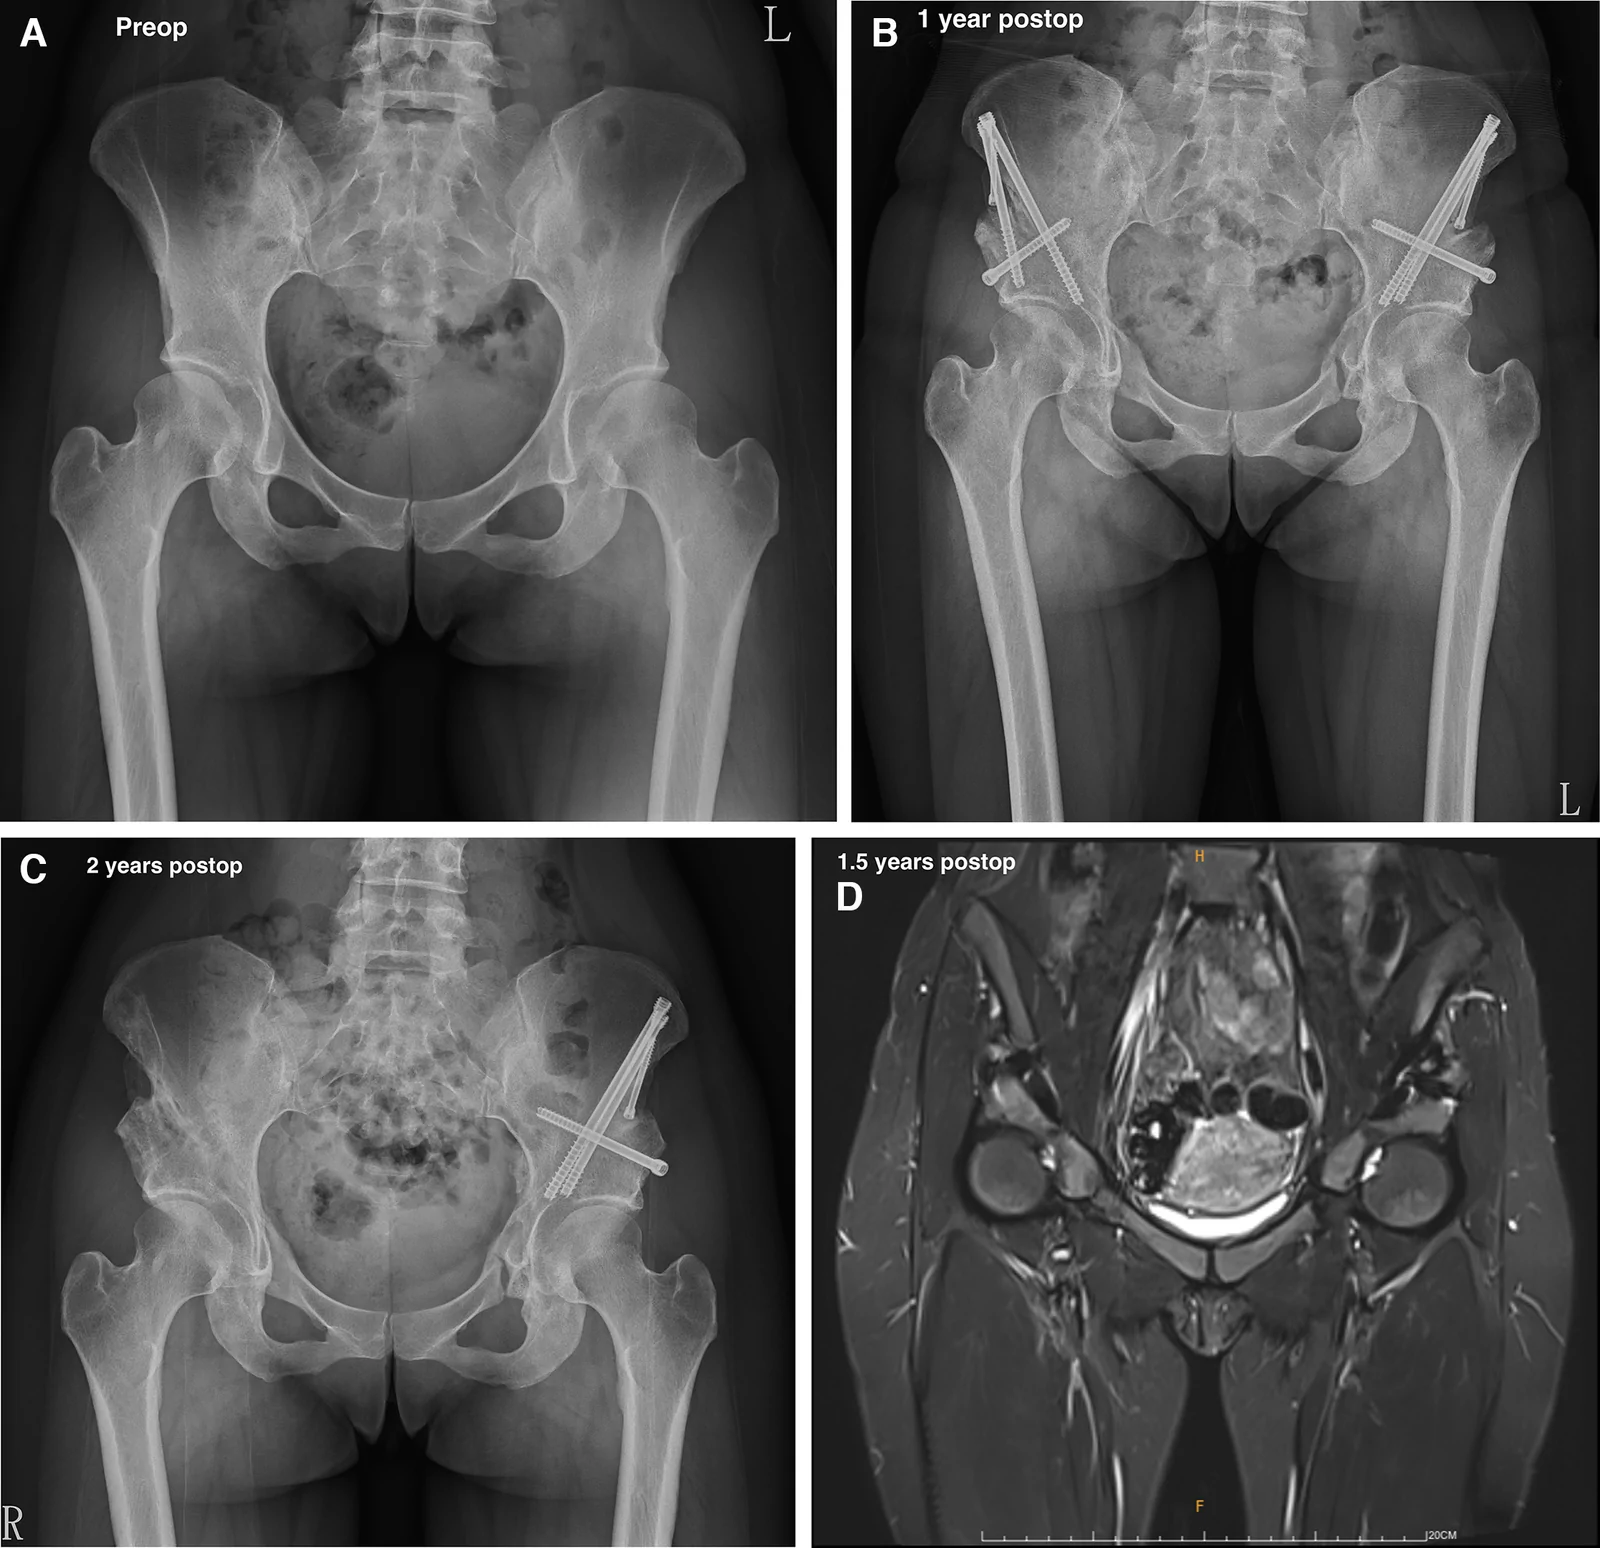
Sequential imaging of a 33-year-old female with bilateral symptomatic hip dysplasia who underwent staged bilateral periacetabular osteotomy (PAO). a) Preoperative weightbearing anteroposterior pelvic radiograph demonstrated bilateral hip dysplasia with lateral centre-edge angle (LCEA) values of 15.7° (left) and 19.5° (right). b) The patient underwent left PAO followed by right PAO five months later. One-year postoperative radiograph demonstrated improved femoral head coverage with LCEA values of 37.5° (left) and 36.4° (right), as well as delayed union of the left superior pubic ramus osteotomy site. c) Two-year postoperative radiograph demonstrated persistent discontinuity of the left superior pubic ramus osteotomy site, consistent with nonunion, with minimal interval change. d) T2 short tau inversion recovery MRI obtained 1.5 years postoperatively confirmed absence of cortical bridging at the left superior pubic ramus osteotomy site.
